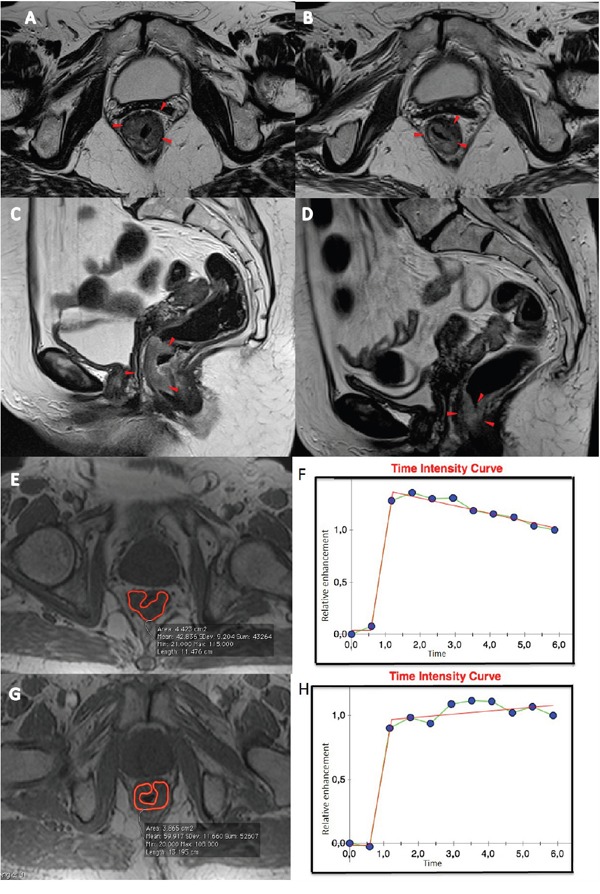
Patient n. 68: T2-weighted images in axial A and sagittal C plan before B the treatment and after treatment D. The morphologic images (A and C) before CRT, showed heterogeneous irregular thickening along the rectal wall spreading into the perirectal fat (A and C, arrowheads). After CRT, a hypointense area with thickening of the rectal wall and straining into perirectal fat (B and D, arrowheads) is observed. Median Time intensity curve of the volume of interest E and G, before F and after treatment H are shown. These curves showed different contrast enhancement, with a ΔSIS value of 39.54% classifying the patient as responder.
